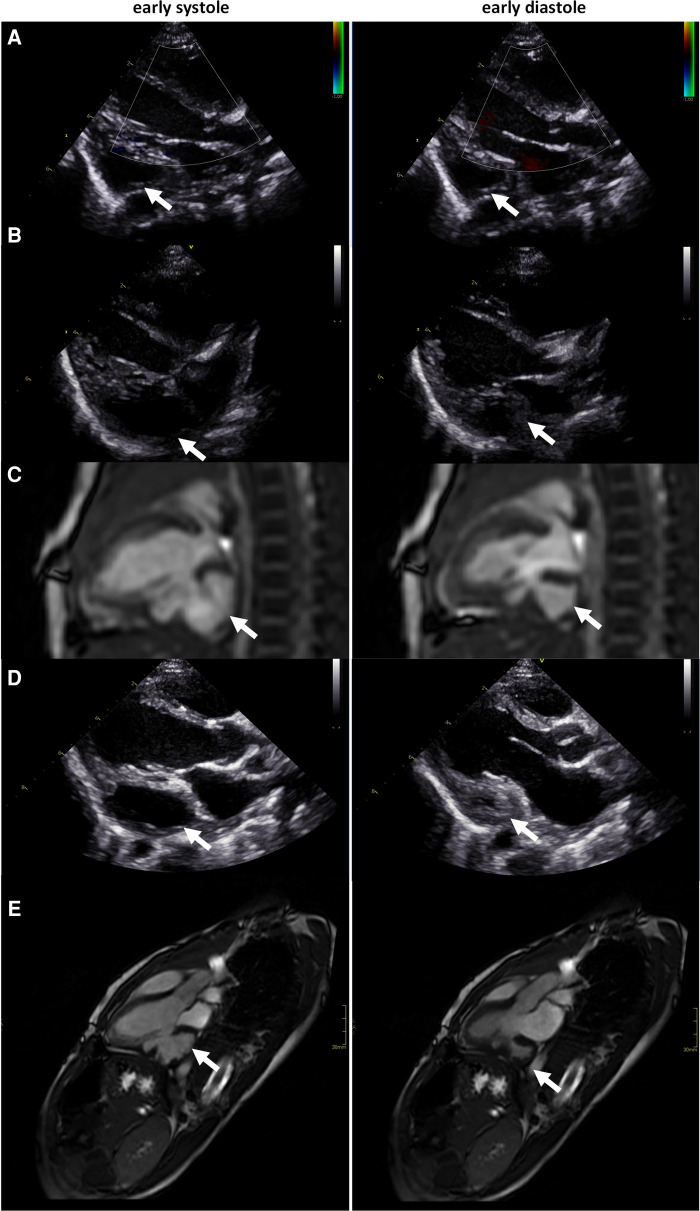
(A) Echocardiography at enrollment: (LVIDd/LVIDs: 2.1 cm/1.9 cm, FS 10%, LVEF 22%), (B) echocardiography at 14-day follow-up (LVIDd/LVIDs: 2.0 cm/1.5 cm, FS 27%, LVEF 55%), (C) CMR at 14-day follow-up (LVEDV: 10 ml, LVESV: 5 ml, LVEF 51%), (D) echocardiography at 4-year follow-up (LVIDd/LVIDs: 3.4 cm/2.0 cm, FS 40%, LVEF 73%), and (E) CMR at 4-year follow-up, displaying an ALS inferior of the left ventricle, determined to be an aneurysm (LVEDV: 40 ml, LVESV: 10 ml, LVEF 75%). CMR, cardiac magnetic resonance; ALS, aneurysm-like structure. LVIDd and LVIDs indicate left ventricular internal diameter end diastole and end systole, FS: fractional shortening, LVEF: left ventricular ejection fraction, LVEDV: left ventricular end diastolic volume, LVESV: left ventricular end systolic volume.
